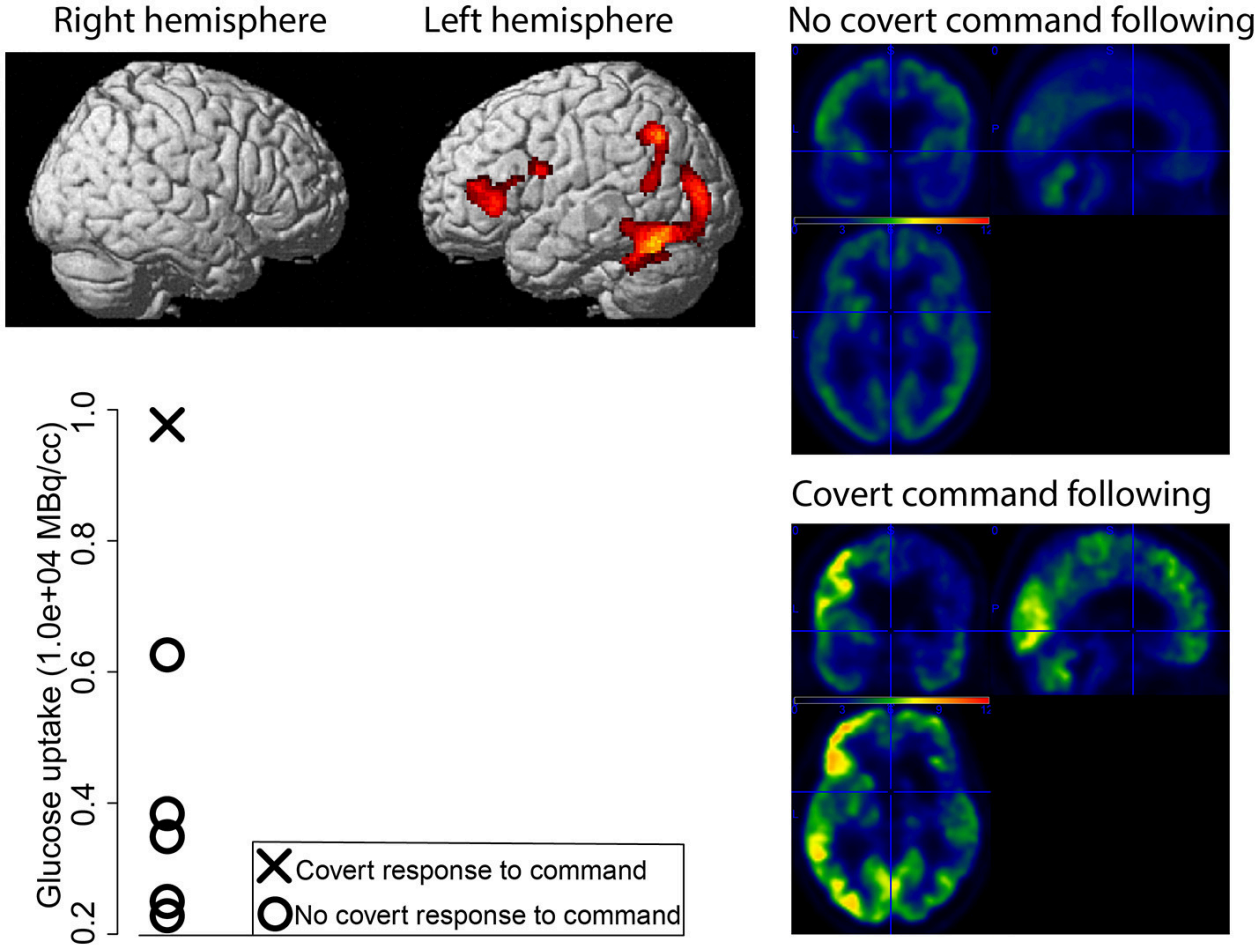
Preserved glucose metabolism (in red-yellow) as measured with FDG-PET for the MCS– patient with signs of “covert command following” compared to patients with a FDG-PET indicative of MCS without signs of “covert command following” (top left). Mean glucose uptake of the more significant cluster (in MBq/cc) for every patient (bottom left, patients with a MCS FDG-PET in absence of “covert command following” represented with circles, the MCS– patient who did show signs of “covert command following” represented with a cross). Average standardized uptake value for the patients without “covert command following” (right top), and the standardized uptake value for the patient with “covert command following” (bottom right).
